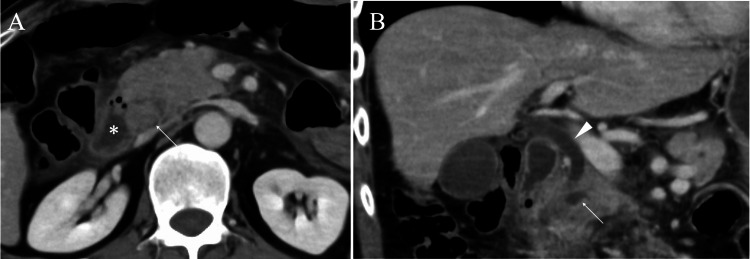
CT findings. (A) Axial view CT showing a presumed pancreatic head tumor (arrow) adjacent to the duodenum (asterisk). (B) Coronal view CT showing pancreatic (arrow) and bile (arrowhead) duct dilatation.
